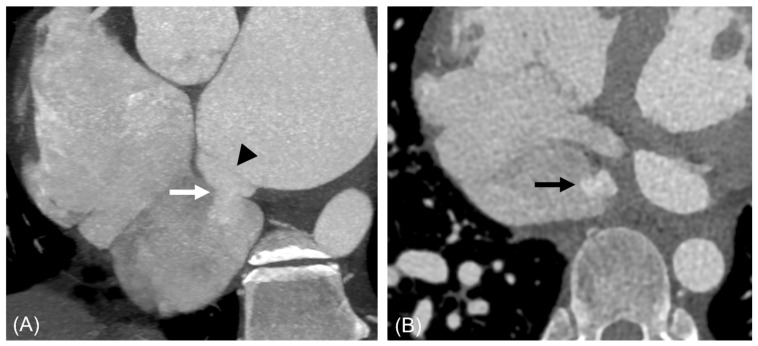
A 42-year-old man with saccular type ILAD communicating with IVC. Short-axis image (A) shows saccular diverticulum demarcated from LA by a membranous structure (arrowhead) and shunt (white and black arrows) below IVC valve (A,B). The shunt size measured 8 mm and was not visualized on transthoracic echocardiography. Abbreviations: ILAD, inferior left atrial diverticulum; IVC, inferior vena cava, LA, left atrium.
